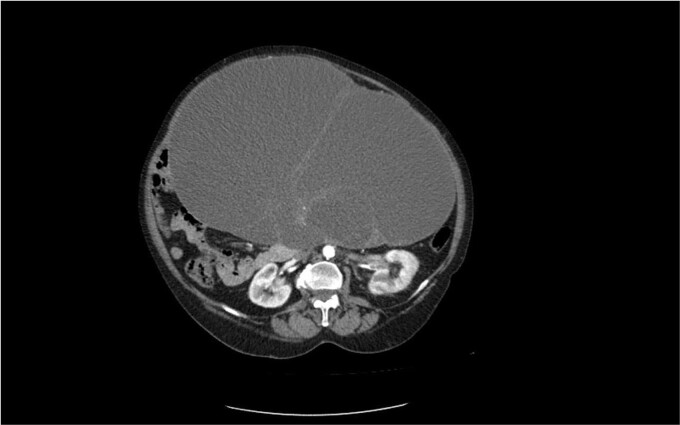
CT image of a mucinous cystadenoma of the left ovary.
